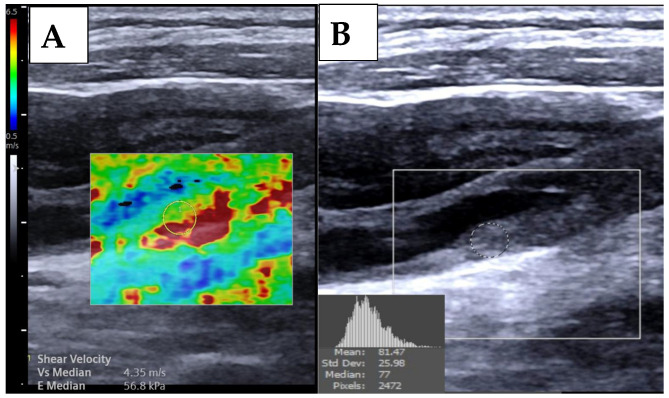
Measurements of ultrasound 2D-SWE (A) and grayscale median (B) from the carotid plaque.
